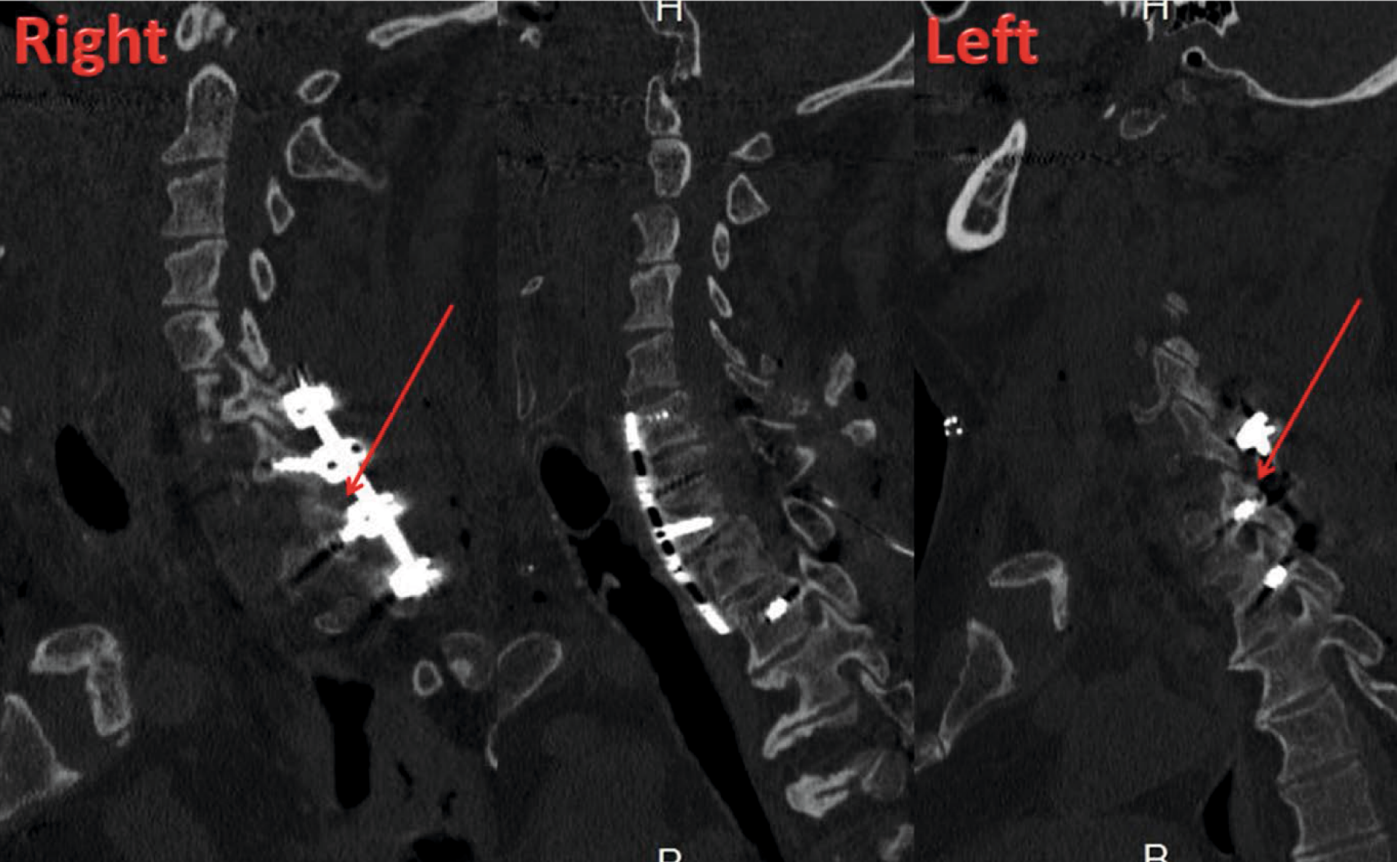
Postoperative CT scan shows complete reduction of cervicothoracic dislocation.
